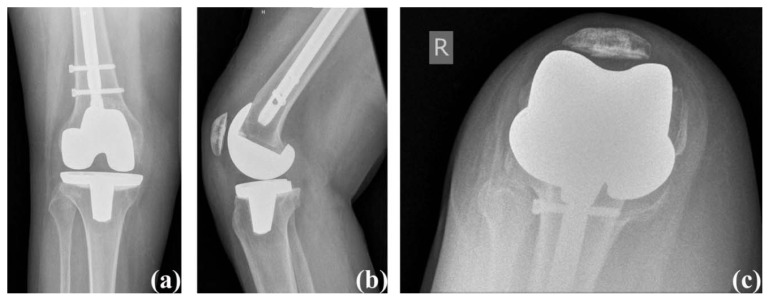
Case #1 post-operative radiographs: (a) anterior posterior view, (b) lateral view, and (c) sunrise view.
